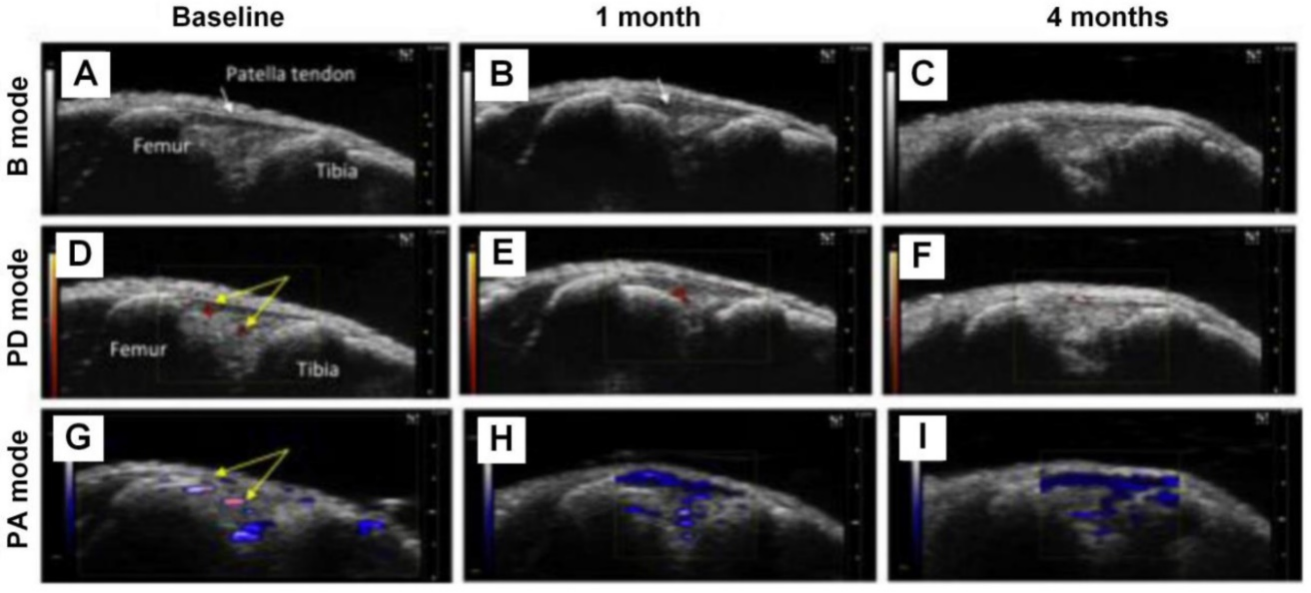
DMM knee joints at different stages being visualized with B mode ultrasound, PD mode ultrasound, and PA imaging, respectively. (A)-(C), B mode ultrasound images of the knee joints at baseline, 1 month, and 4 months, respectively. (D)-(F), PD mode ultrasound images of the knee joints at baseline, 1 month, and 4 months, respectively. (G)-(I), PA images of the knee joints at baseline, 1 month, and 4 months, respectively. Figures adapted with permission from Ref 80. Copyright © 2018, Elsevier.
